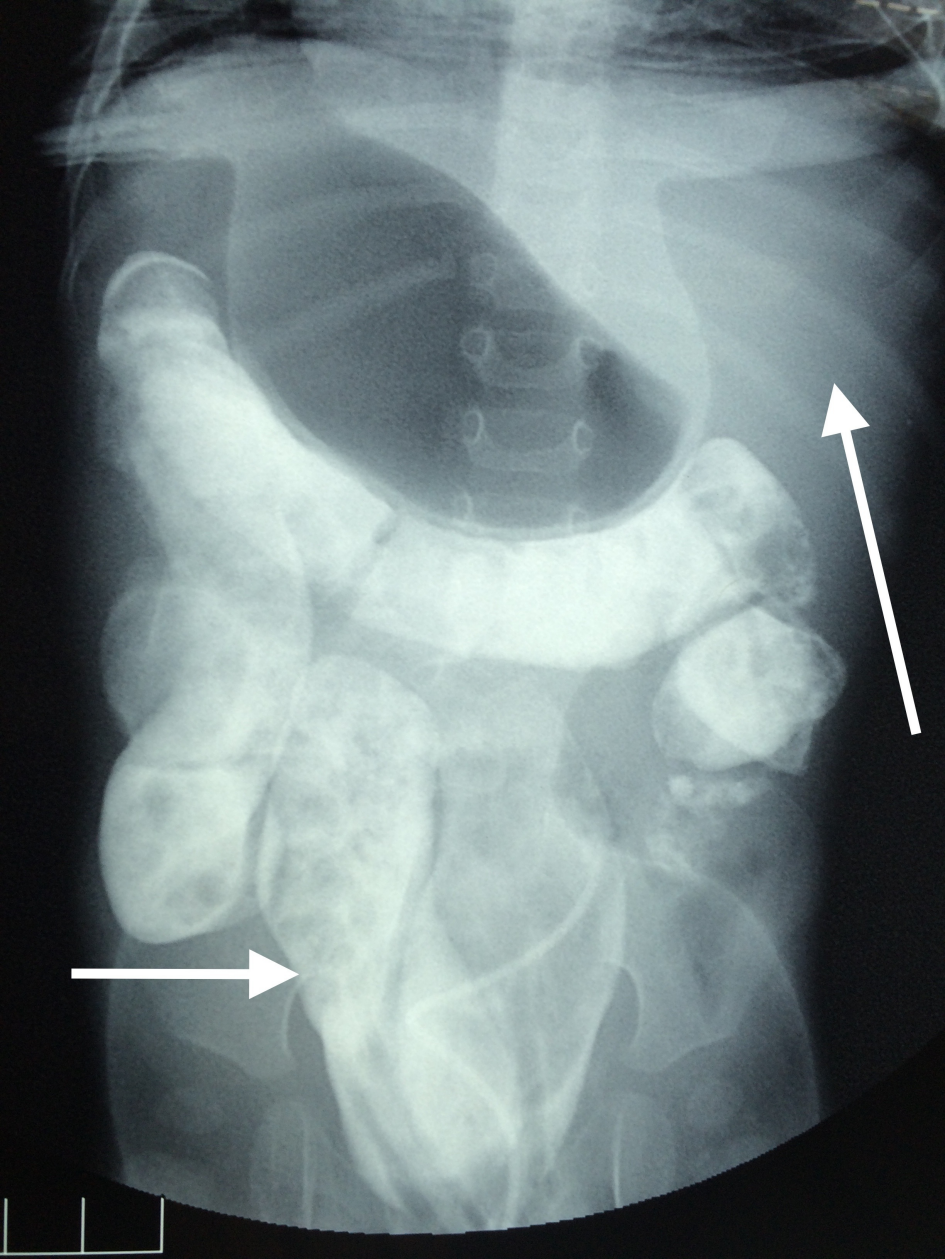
Contrast enema abdominal radiograph showing duplicated sigmoid colon (small arrow), liver on the left side (large arrow)
